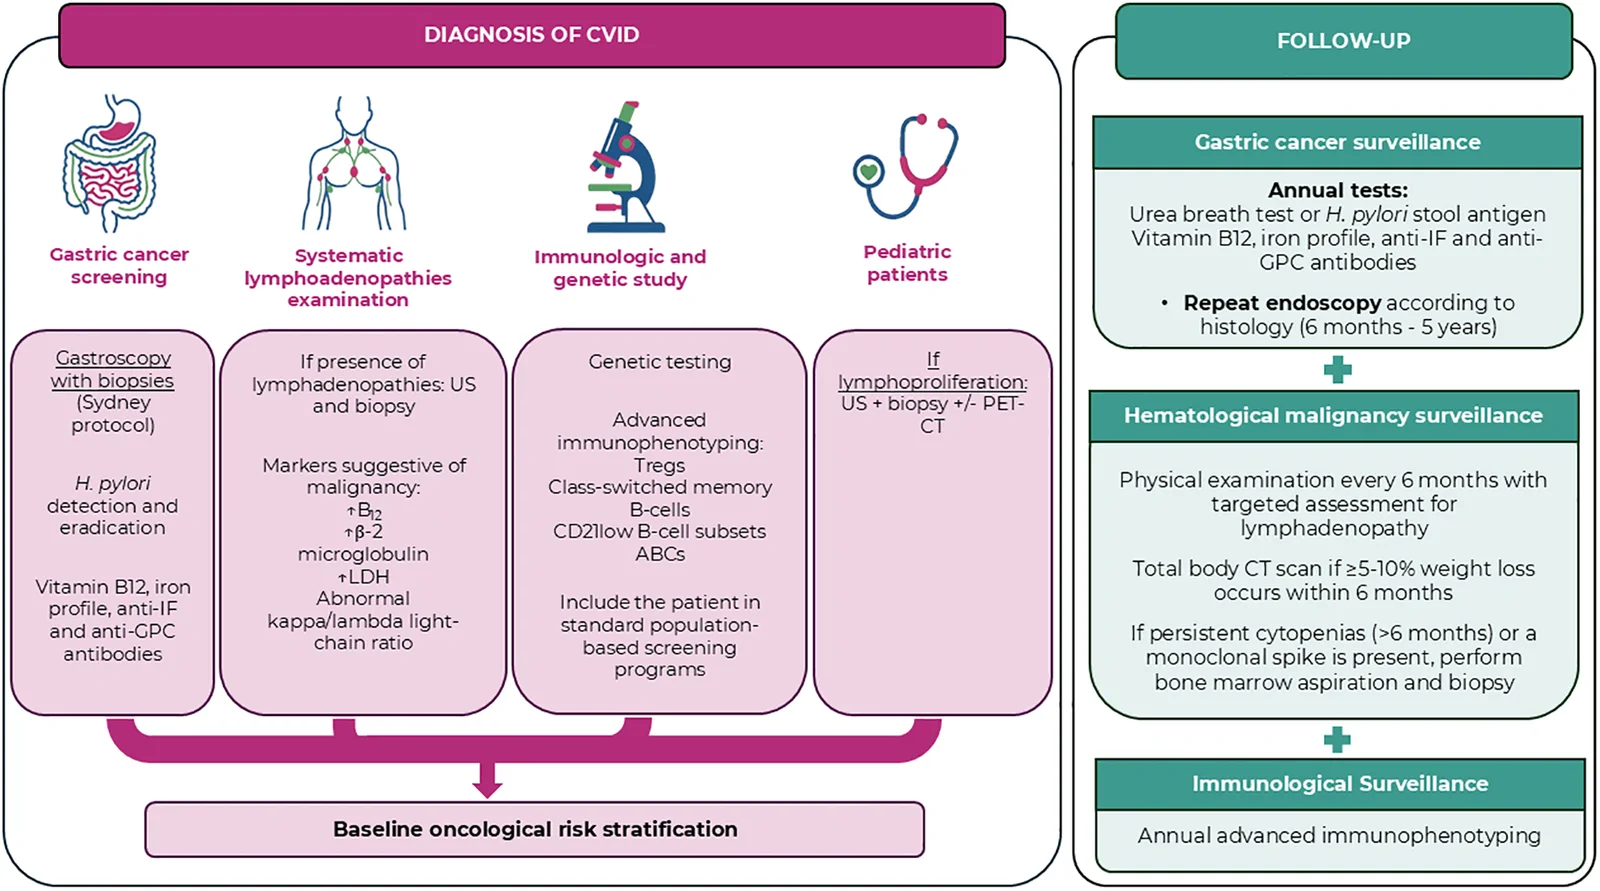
Consensus-based recommendations for diagnosis and follow-up of malignancies in patients with CVID. ABCs, atypical B cells; anti-GPC, anti-gastric parietal cell; anti-IF, anti-intrinsic factor; CT, computed tomography; CVID, common variable immunodeficiency; H. pylori, Helicobacter pylori; LDH, lactate dehydrogenase; PET-CT, positron emission tomography-computed tomography; Tregs, regulatory T cells; US, ultrasound.
